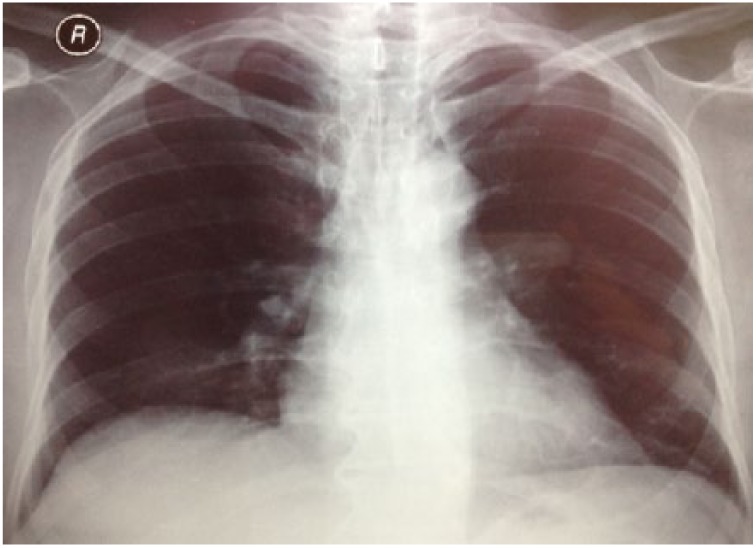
The control chest x-ray before anti-TNF treatment (March 2010).
